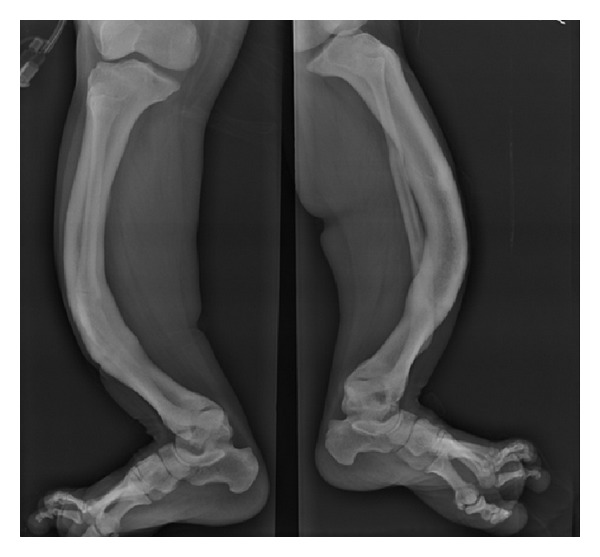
X-ray showing bowing of long bones.
